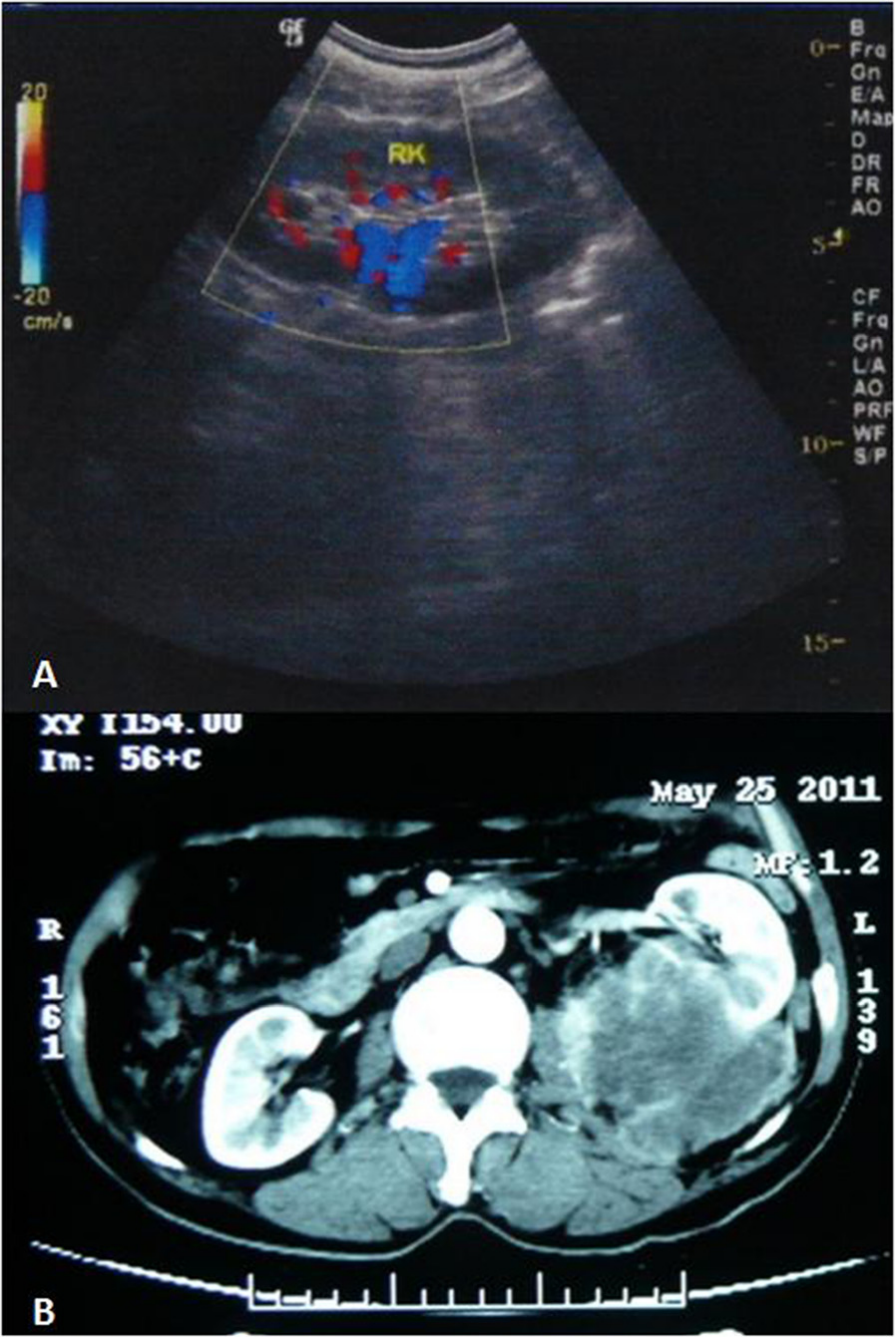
Radiological features of the tumor. (A) Ultrasound examination showed a space-occupying mass of low echogenicity within irregular margin. (B) Computed tomography showed a large mass in the left kidney.
